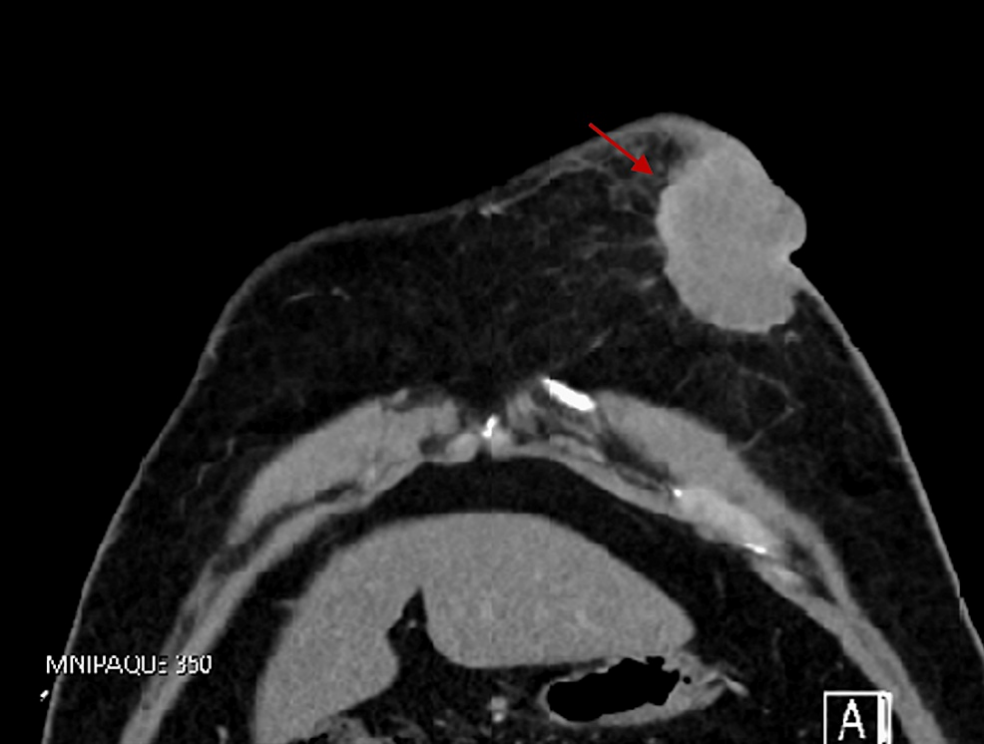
CT thorax showing a heterogeneous soft tissue mass
